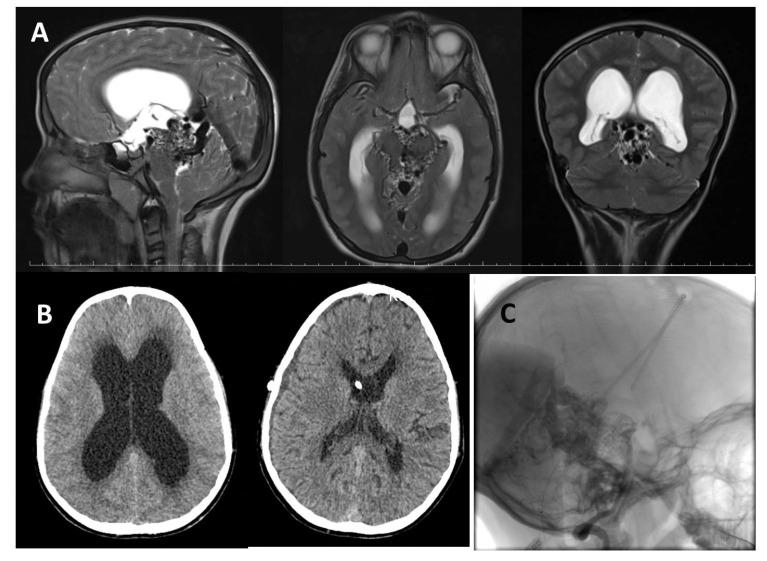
A) T2 weighted MR scans showing a mesencephalic AVM that caused hydrocephalus with obstruction of aqueduct in sagittal, axial and coronary planes. B) A control CT showing the enlarged lateral ventricle with transependymal edema (left), and the collapsed ventricles following VP shunt procedure, with tip of the ventricular catheter (right). C) A DSA view perimesencephalic AVM in lateral plane, with intraventricular catheter.
